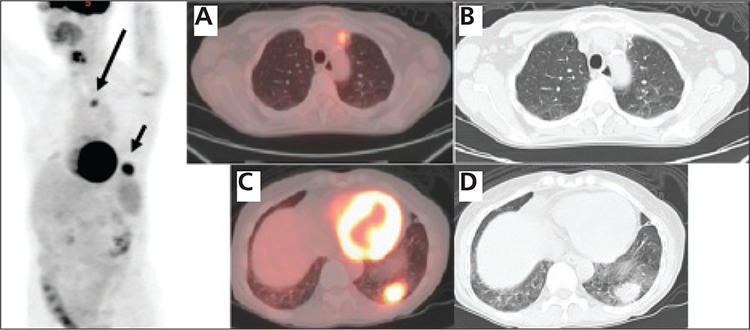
After biopsy of the left lower lobe lesionrevealed NHL, the initial staging PET/CT shows the increased FDGuptake associated with these pulmonary opacities in the left uppermedial chest (large arrow), and in the left lower lateral chest (smallarrow) on the 3D PET image. The fused axial image (A) and axial CTslice on lung window (B) demonstrate the increased FDG uptakeassociated with the opacity in the left upper lobe, with SUV max of5.2, which correlating the upper medial FDG uptake on 3D PETimage. The fused axial image (C) and axial CT slice (D) show the FDGavid parenchymal lesion in the left lower lobe measuring 2.9x2.2 cmwith SUV max of 10.2, correlating to the lower lateral FDG uptakeon 3D PET image. No other site of abnormal increased FDG uptakeis noted on the rest of the whole body PET images to suggest othersites of extranodal or nodal lymphomatous involvement
